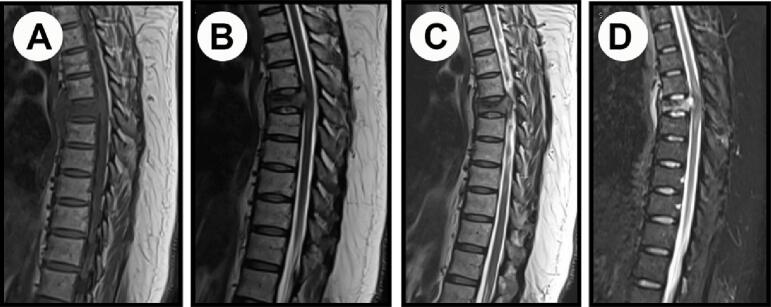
MRI spine of the patient showing a pathological compression fracture and a paravertebral, paravertebral, and retro-vertebral intraspinal mass that is hyperintense on T2 STIR sequence (d) and hypointense on T1 sequence (a), T2 sequence (b), and T2 TSE sequences.
